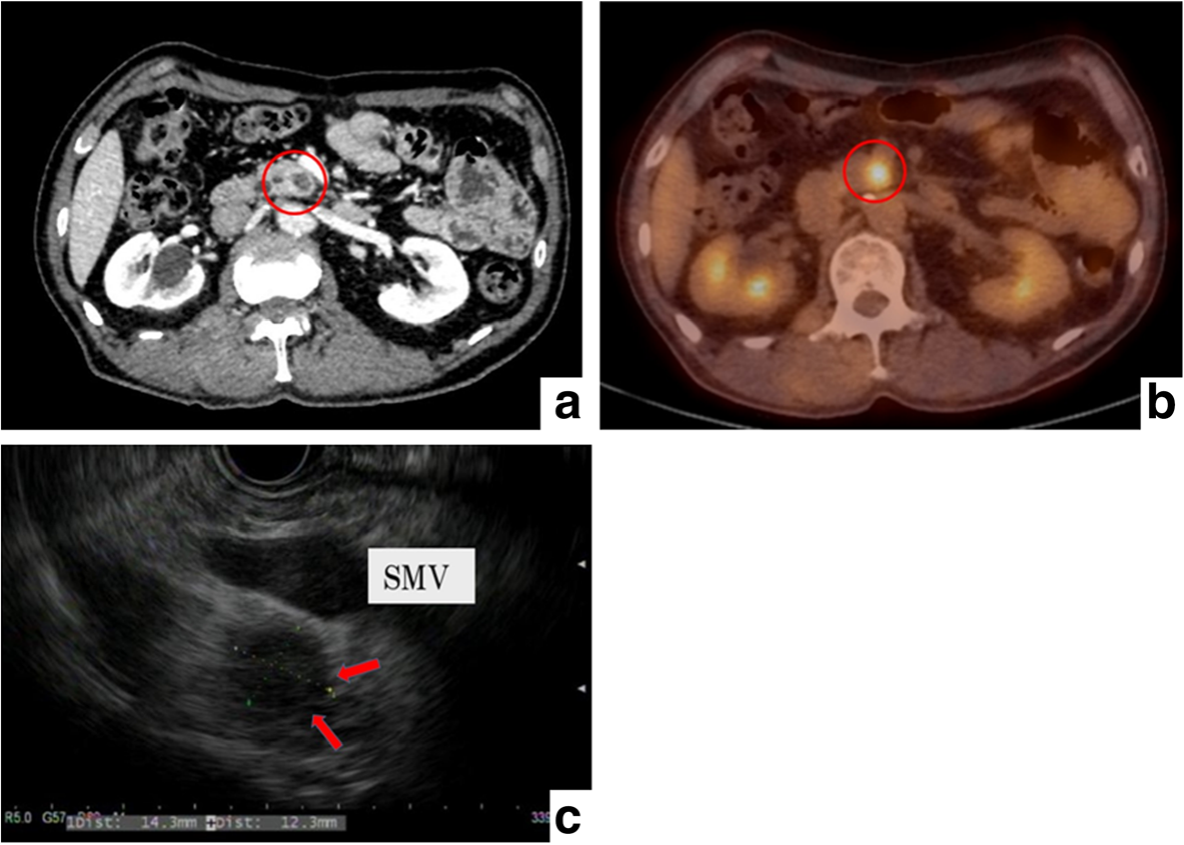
Radiological and endoscopic ultrasonographic findings in case 2. a CECT revealed an 8-mm hypovascular tumor in the uncus of the pancreas. b PET-CT showed FDG accumulation (SUVmax 5.6) corresponding with the pancreas tumor. c EUS detected a 14 × 12-mm hypoechoic lesion in the uncus, but we could not perform EUS-FNA because the superior mesenteric vein was located in the puncture line
